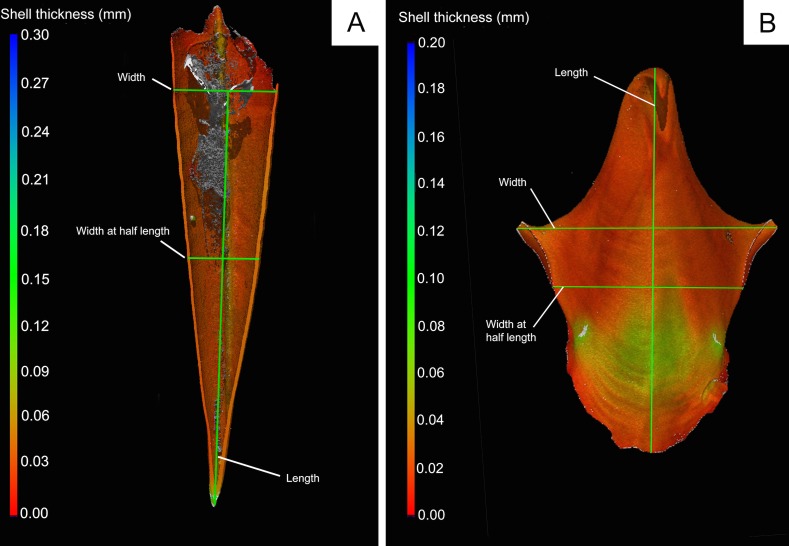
Three Dimensional CT renderings of A. *Styliola subula* (in cross section) and B. *Cavolinia inflexa*. Colouring indicates assessment of shells thickness at a particular point via standard “wall-thickness” analysis with VGStudio MAX, with thickness scales given to the left of each panel. Also indicated, as green lines are dimension measurements taken from the CT renderings. The grey area inside the *S*. *subula* is organic material and the lack of colouring indicates that it has been excluded from the thickness determination.
